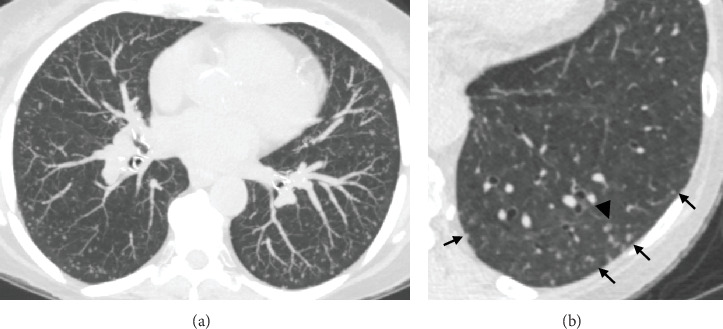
Companion case of multiple stable micronodules, presumed DPM, in a 65-year-old female. (a) Axial noncontrast chest CT image reconstructed with a 5-mm slice thickness using MIP shows bilateral innumerable and diffuse micronodules in clusters predominantly near the pleural surfaces. (b) Axial thin slice image (0.6-mm slice thickness) zoomed in the left lower lobe shows the micronodules with variable size, shape, and densities (arrows), one of which with central lucency (arrowhead).
